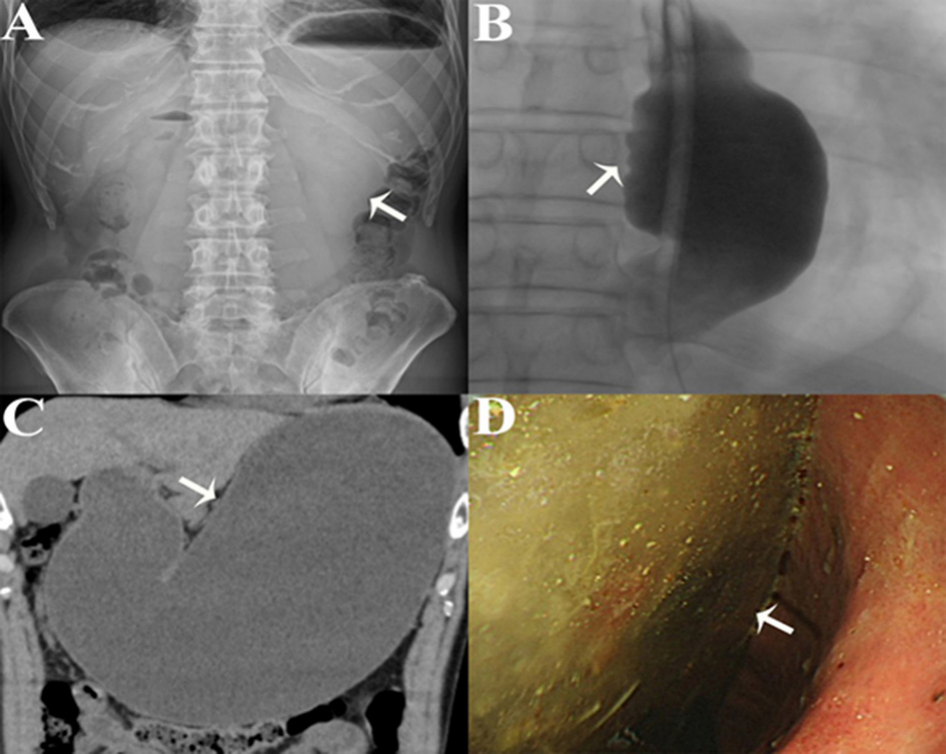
(A) An abdominal X-ray suggests gastric distension. (B) The X-ray iodine contrast radiography image shows esophageal distension. (C) Abdominal CT revealed gastric dilatation without any gastric or intestinal obstruction. (D) Esophagogastroduodenoscopy reveals an abundance of food residue, and no obstructing lesion at the pylorus.
